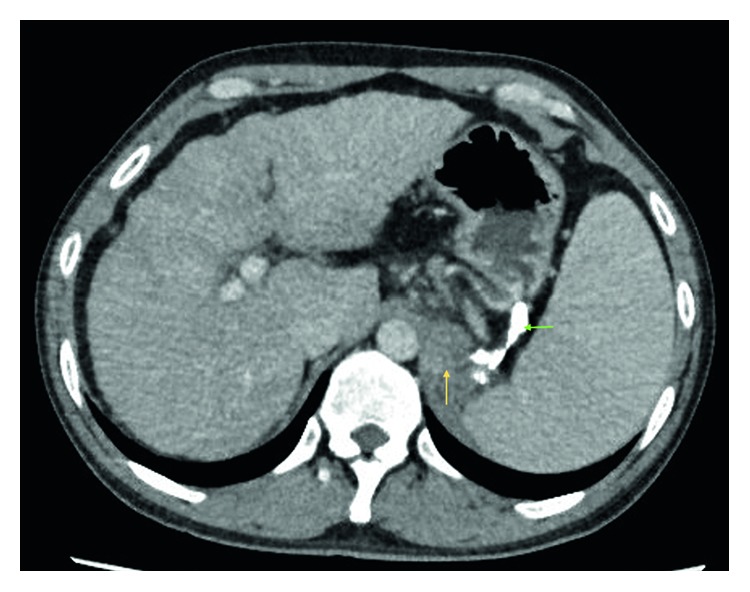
Computed tomography view of the abdomen (in axial view) after completion of antibiotics. The previously seen retrogastric abscess has completely resolved leaving behind a small area of scarred tissue (yellow arrow). The residual N-butyl-2-cyanoacrylate in the perigastric region is still visible (green arrow).
